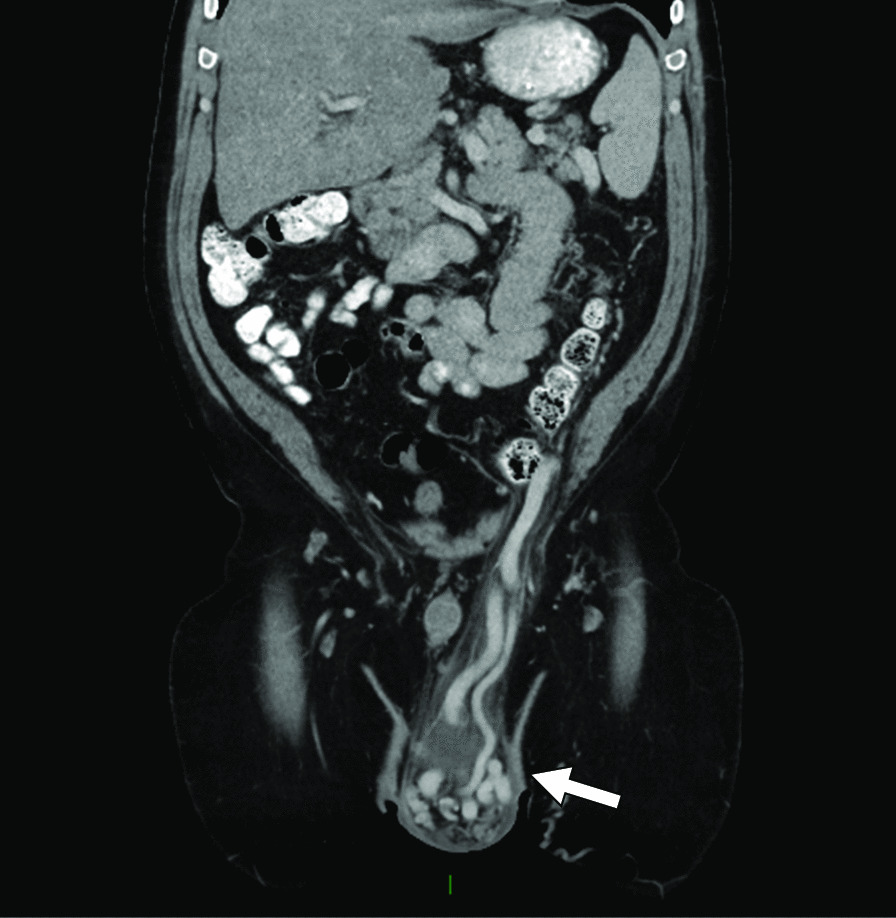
Coronal contrast enhanced CT image showing dilated left spermatic veins (arrow)
